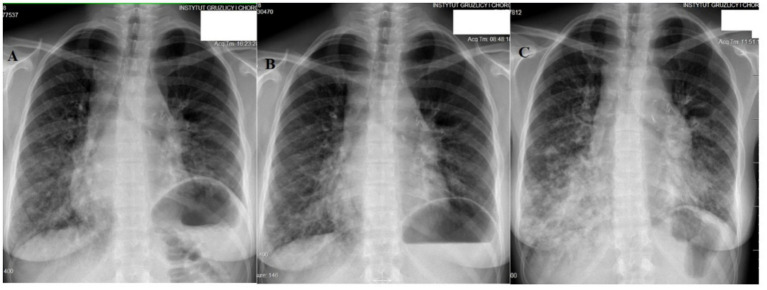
Patient No. 1’s chest X-ray examinations. (A) Posteroanterior (PA) chest X-ray image at the time of treatment initiation shows bilateral opacities, mostly in the middle and lower fields of the right lung, and smaller quantities in the left lung. The outline of the mediastinum and pulmonary hila is widened, and the left diaphragm dome is elevated. (B) PA chest X-ray image after 1 year of sirolimus treatment shows a distinct reduction in bilateral lung opacities. The outline of the mediastinum and pulmonary hila is widened. The left diaphragm dome is elevated. (C) PA chest X-ray image after 5 years of sirolimus treatment (with significant treatment-free periods due to non-adherence) shows massive, bilateral progression of lung opacities, predominantly in the lower and middle fields of the right lung.
